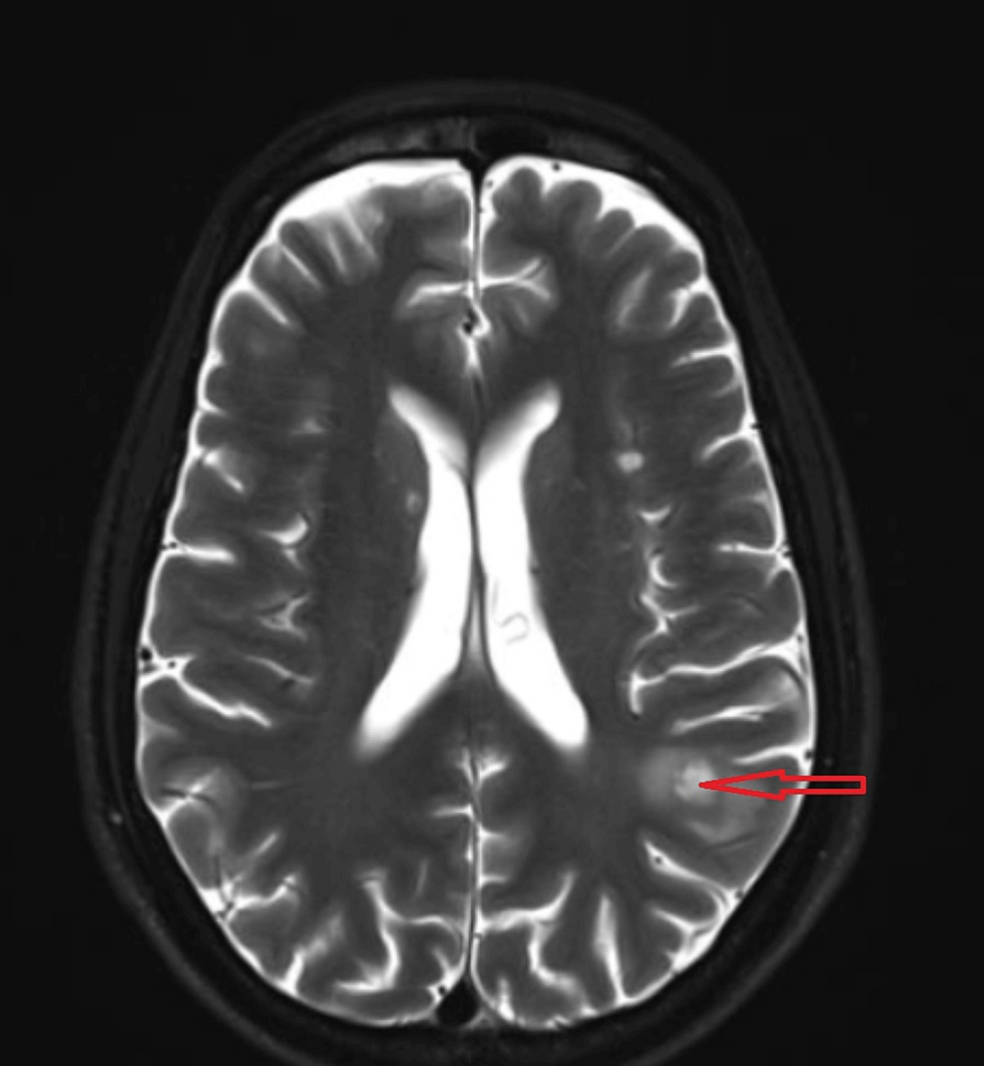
MRI of the brain showing ring-enhancing lesions in the brain parenchyma.
